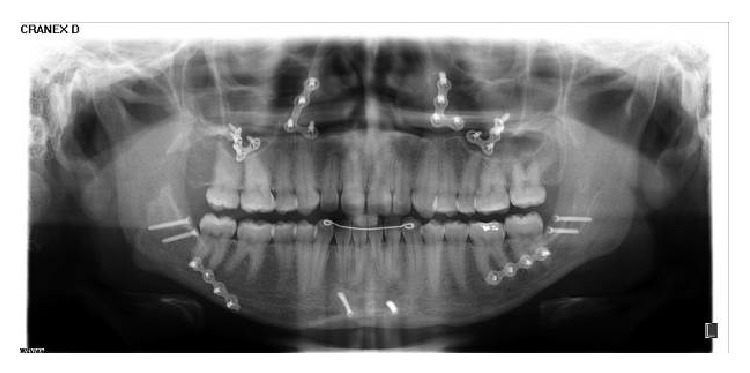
7-year posttreatment panoramic radiograph.
